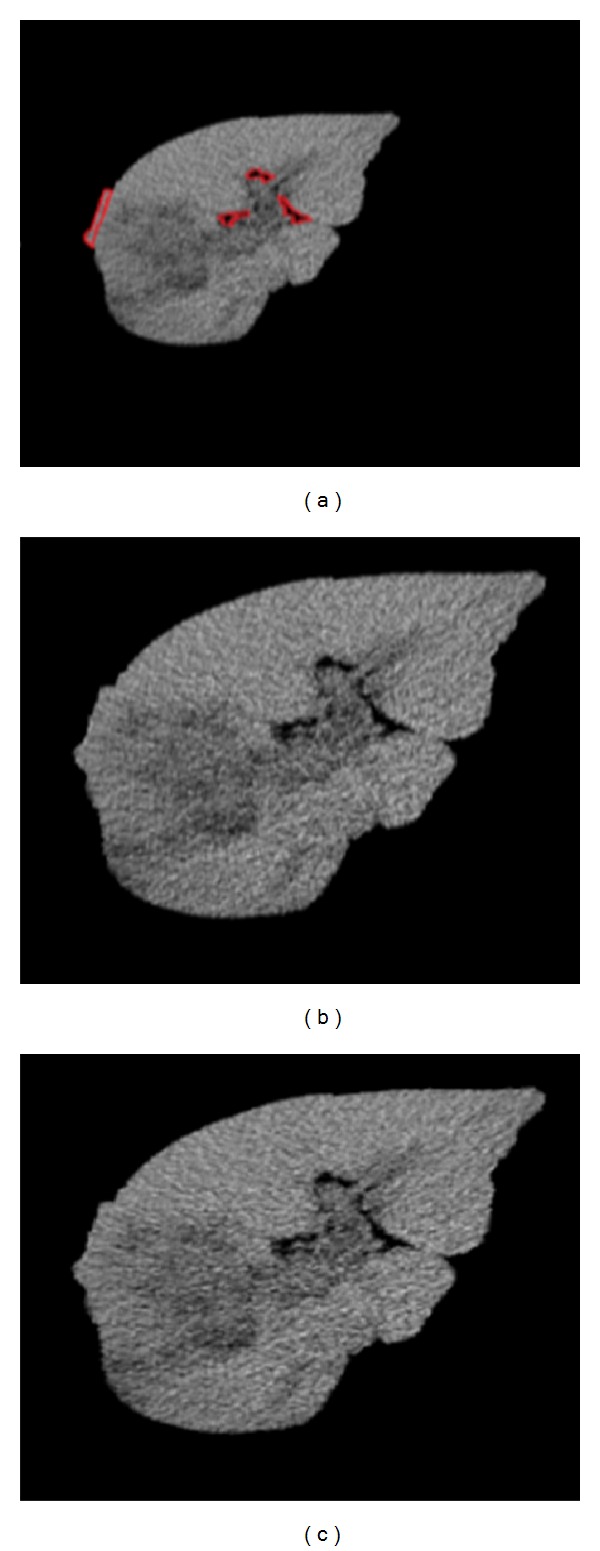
Preprocessing for liver CT images. (a) Liver region. (b) Normalization result. (c) Histogram equalization result.
